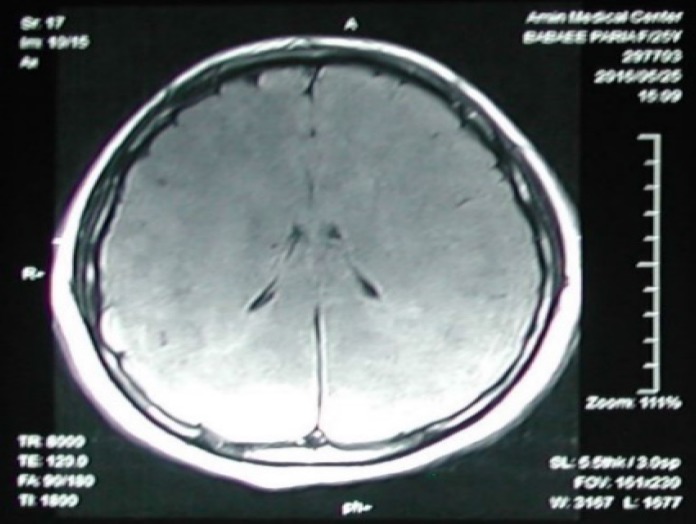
Axial FLARE image in magnetic resonance imaging (MRI) of brain shows diffused brain edema (1st day of coma)
